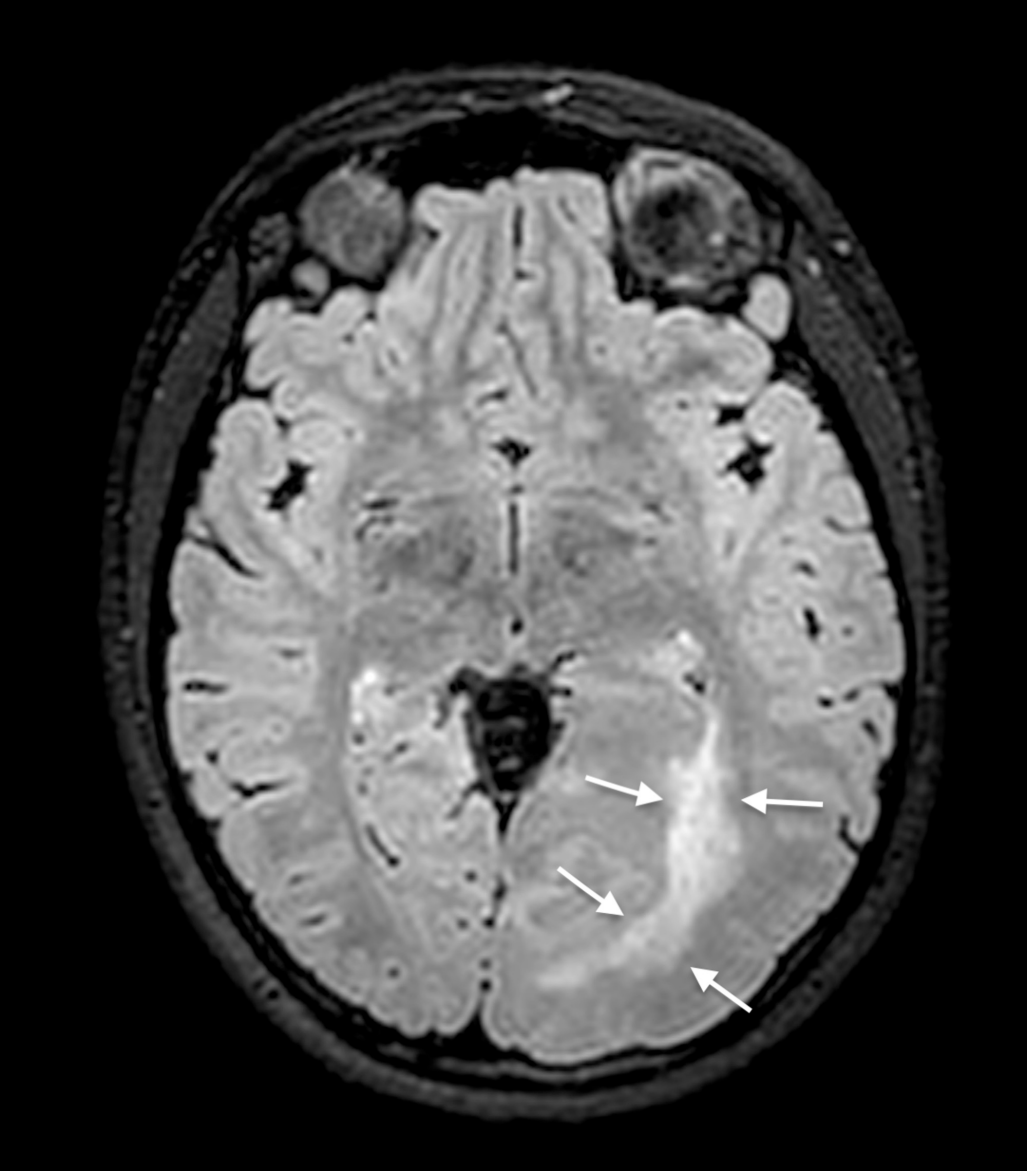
Three-dimensional FLAIR sequence with axial reformatting Increased signal intensities around the left occipital horn consistent with astrogliosis (white arrows). FlAIR: fluid-attenuated inversion recovery.
